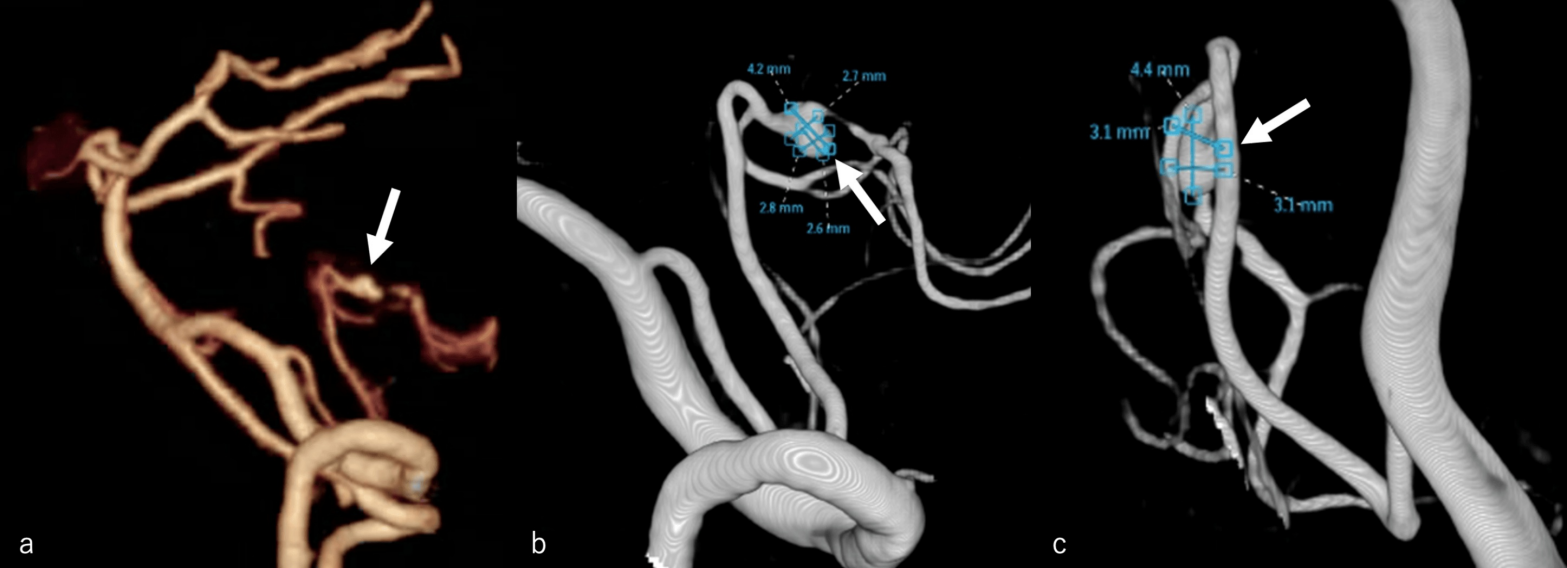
(a) Head computed tomography angiography and (b and c) cerebral angiography revealing a dissecting aneurysm in the telovelar tonsillar segment of the left distal posterior inferior cerebellar artery (b: lateral view, c: frontal view) (white arrow)
